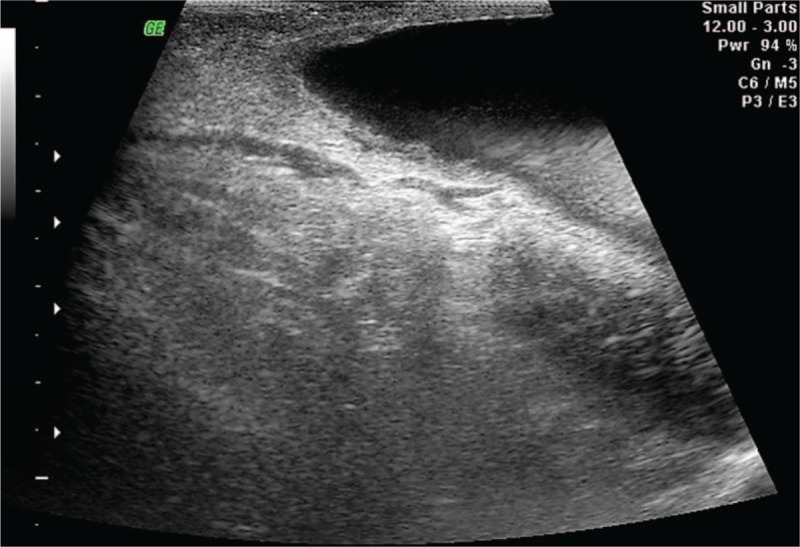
Ultrasonography image of a subcutaneous anechoic, fluid filled mass located near to the right superficial inguinal ring.
